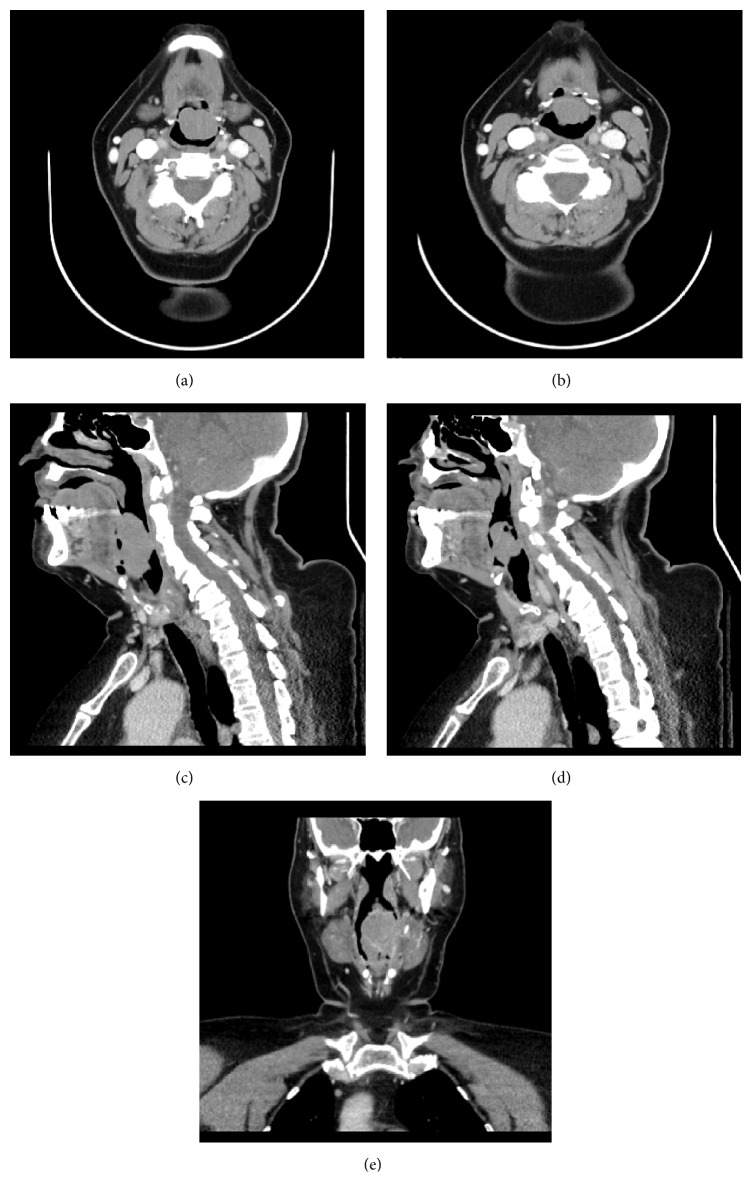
Axial CT neck (a and b), sagittal CT neck (c and d), and coronal CT neck (e), with contrast, show the anteriorly sessile soft tissue mass arising from the laryngeal surface of epiglottis, with relatively stable position. The epiglottis has no submucosal extension.
